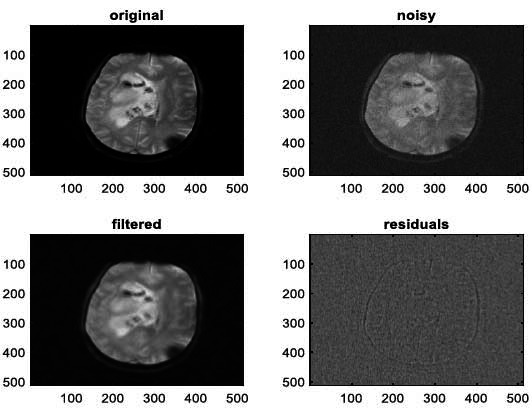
The second example of MRI denoising by applying the proposed image denoising approach.
